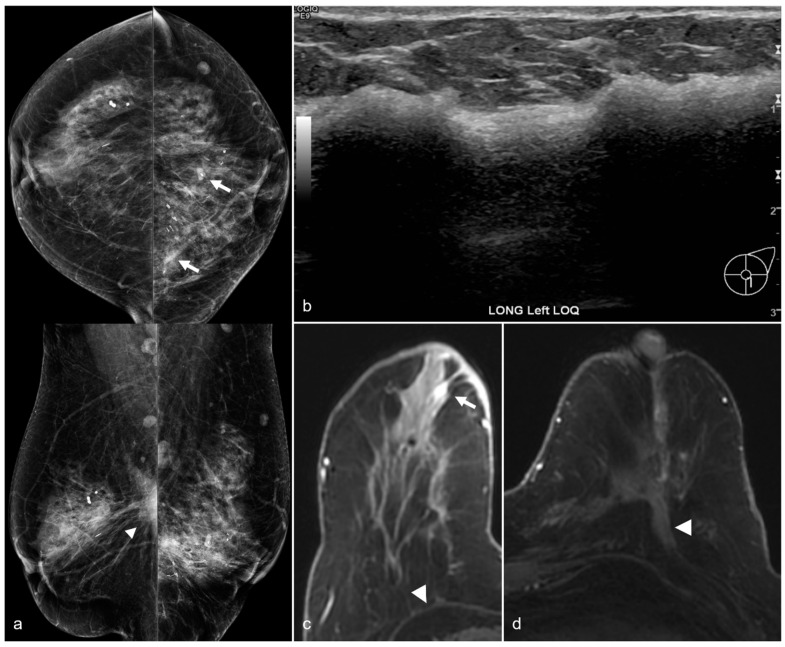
A 79-year-old woman with a history of subglandular silicone implants with a remote history of BES. Mammography (a) demonstrates dense masses in the left breast (arrows) and a right prepectoral asymmetry (arrowhead). Four biopsy clips are also seen in the left breast secondary to prior benign biopsies. Longitudinal left breast ultrasound (b) demonstrates an extensive snowstorm appearance, which limits evaluation of the breasts. T1-weighted post-contrast axial MRI (c) reveals an enhancing 0.3 cm focus in the right central breast (arrow). Subsequent MRI-guided biopsy revealed multifocal LCIS and ALH, and the patient underwent breast conservation therapy. Note the post-BES changes in the bilateral prepectoral regions (arrowheads), which is more pronounced in the left breast (d).
